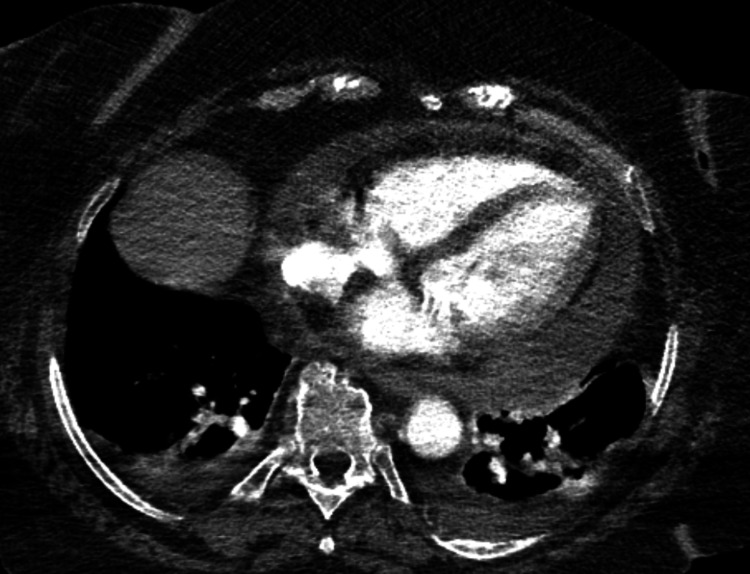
CT chest with contrast showing a large circumferential pericardial effusion CT, computerized tomography.
